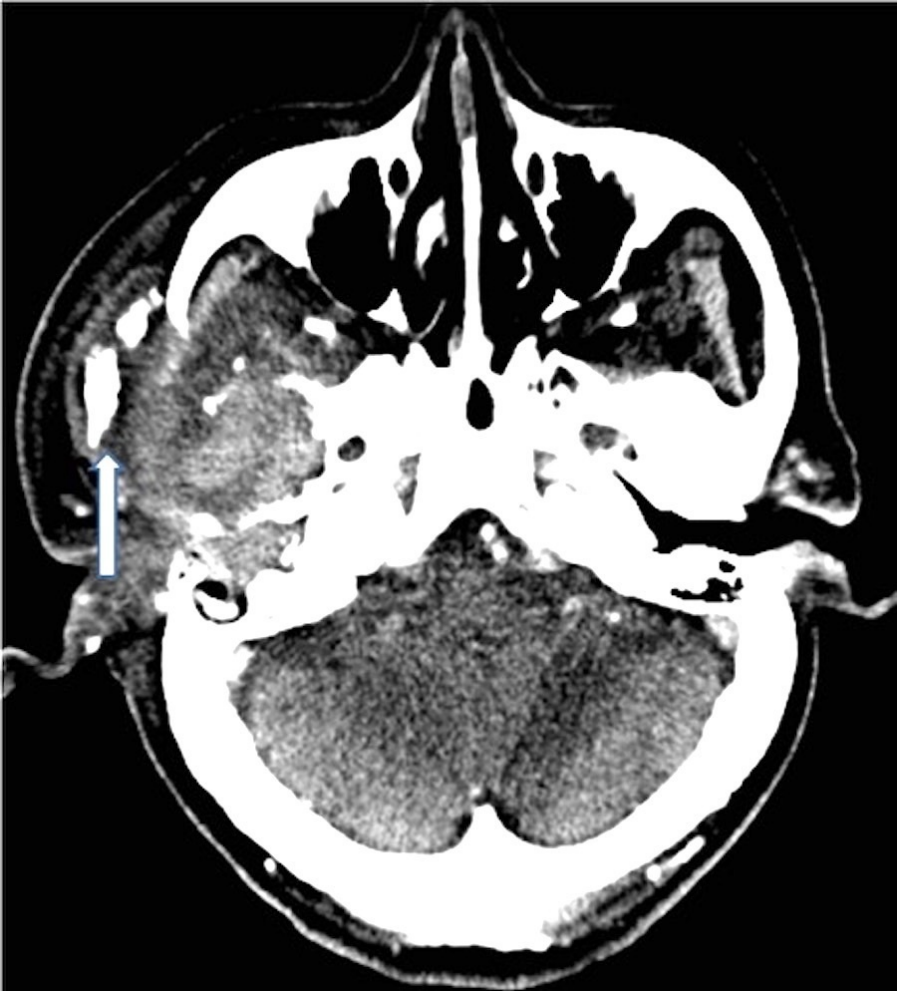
Contrast-enhanced computed tomography of the neck demonstrates the heterogeneous mass involving the right infratemporal fossa. There is contiguous destruction of the petrous temporal bone consistent with osteomyelitis. Note the bulky dystrophic calcifications lateral to the zygomatic arch (arrow).
